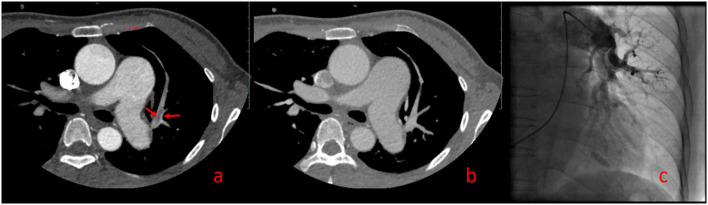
Pulmonary insufficiency artifacts (PIA, Supplementary Video 5). A 39-year-old woman with pulmonary hypertension underwent dual phase-computed tomography pulmonary angiography. A strip filling defect can be seen in the superior lingular branch of left pulmonary artery (SLBLPA), as shown by the arrow in (a) (pulmonary artery phase); SLBLPA is well displayed, and there is no filling defect in (b) (aortic phase); (c) Pulmonary angiography confirmed that there was no PE in SLBLPA.
